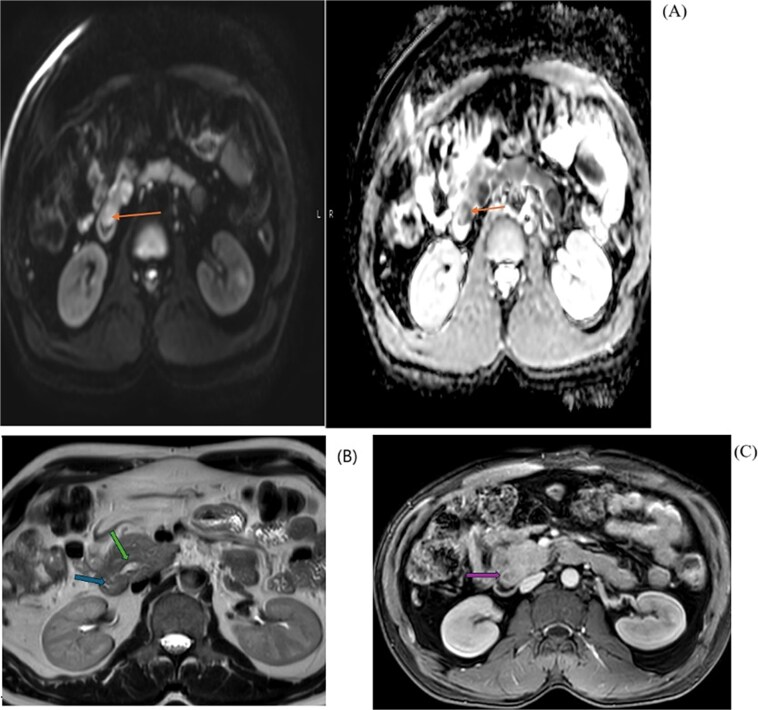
MRI diffusion/ADC map (A) shows restriction pattern of the periampullary lesion reflecting bright signal on diffusion and dark signal on ADC map. MRI axial T2 haste image at the level periampullary region (B) shows rounded isointense lesion. And post IV contrast axial MRI image (C) shows hypo enhancement of the peri-ampullary mass.
